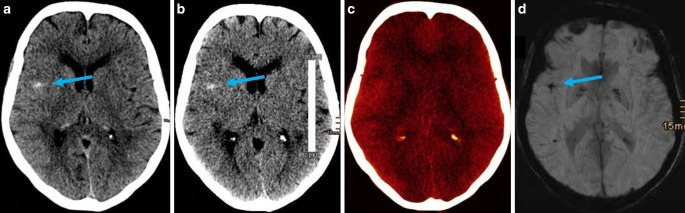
A standard native non-contrast computed tomography (CT) mixed image from TwinSpiral dual-energy CT shows a hyperdensity in the Sylvian cortex on the right side (*blue arrow*) in an acute ischemic stroke patient after endovascular thrombectomy (a). The hyperdensity is also seen in the same location on the virtual non-contrast (VNC) image (b). No hyperdensity is seen on the iodine map (c). Follow-up magnetic resonance image (MRI) (d) showing a susceptibility artifact (*blue arrow*) on a susceptibility-weighted imaging (SWI) corresponding to the hyperdense area seen on the standard mixed and VNC images, but not on the iodine map, thus proving an intracerebral hemorrhage
